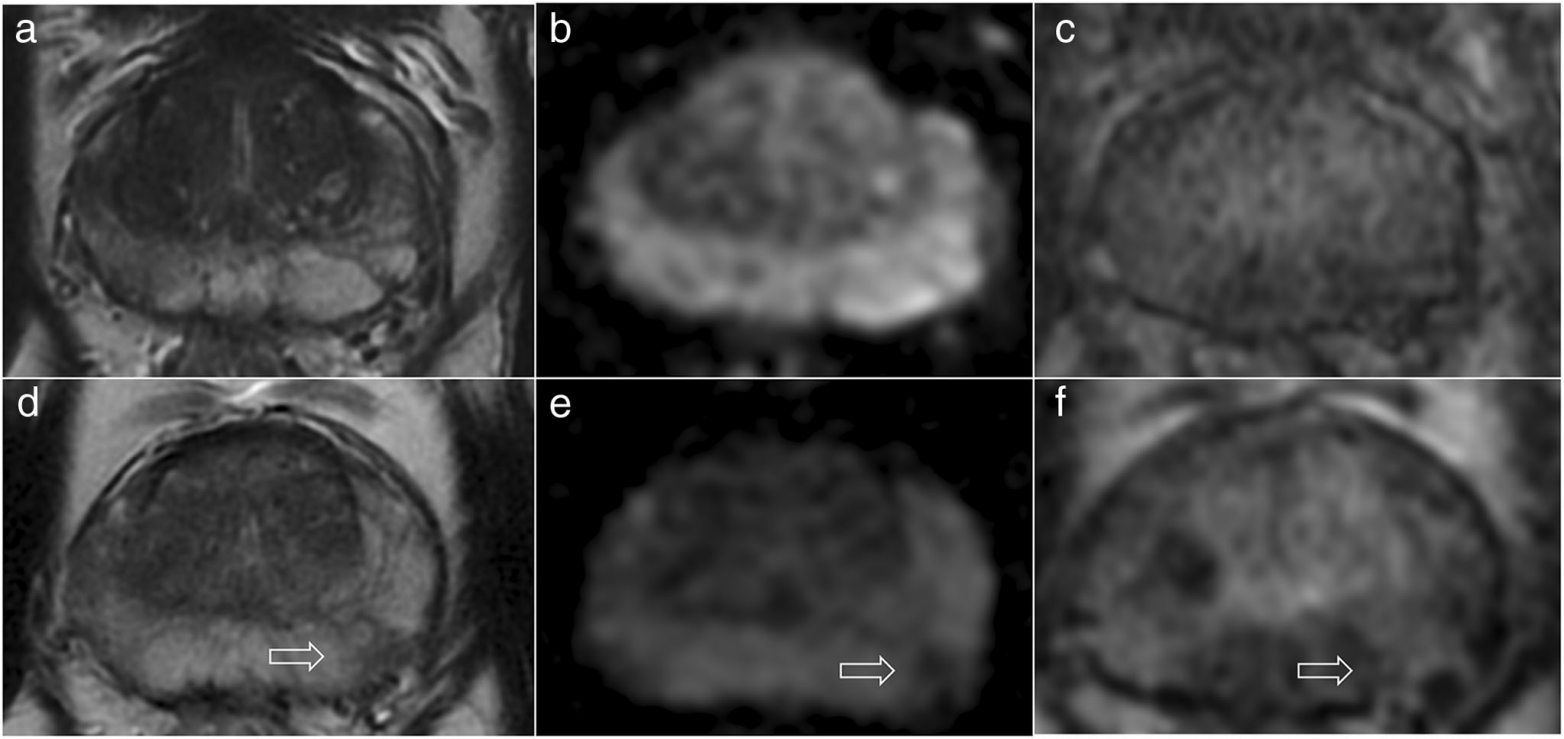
52-year-old patient on active surveillance for Gleason 3 + 3 (1 mm) in the right midgland peripheral zone and a presenting PSA of 6.02 ng/ml (PSA density, 0.12). The first 3-T MRI scan (a–c) did not show any focal lesion but only some patchy diffuse low T2-signal (a) and mild enhancement in the peripheral zone on the right (c) but no restricted diffusion on the ADC map (b). The scan after two years (d–f) revealed a new focal area (arrows) of low T2-signal (d), restricted diffusion on the ADC map (e) and mild enhancement (f) in the left peripheral zone, with a PSA of 8.89 ng/ml (PSA density, 0.18). The PRECISE score was 4 for both radiologists, and targeted biopsy of the area revealed Gleason 3 + 3 (3 mm)
